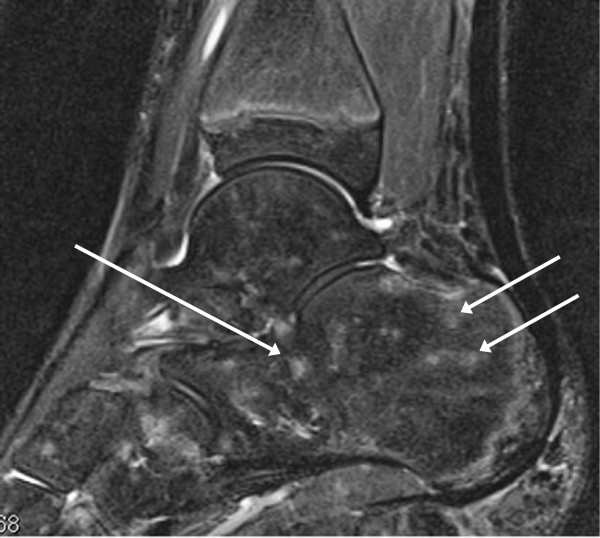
Imaging of the asymptomatic ankle region of an 11-year-old healthy control. A sagittal STIR MRI revealing multiple and patchy signal changes suggestive of foci of haematopoietic red marrow, or of focal bone marrow edema (arrows).
